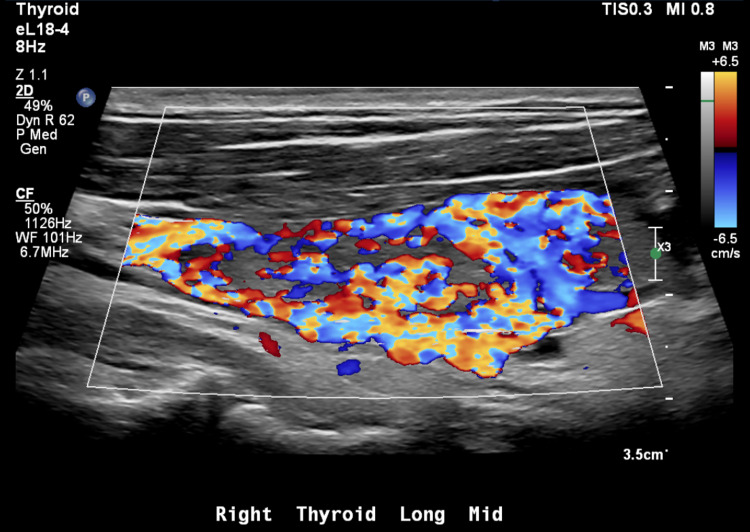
Increased flow of the right thyroid lobe on color doppler ultrasound
